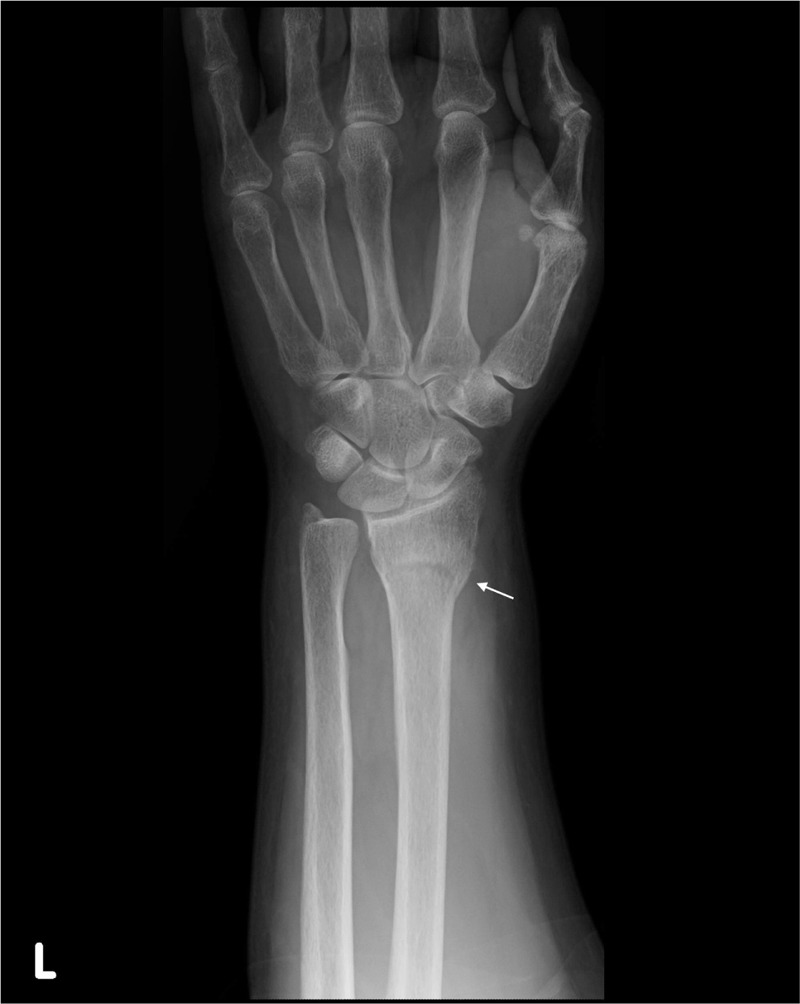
An x-ray showing left distal radius fracture of the patient (arrow).
